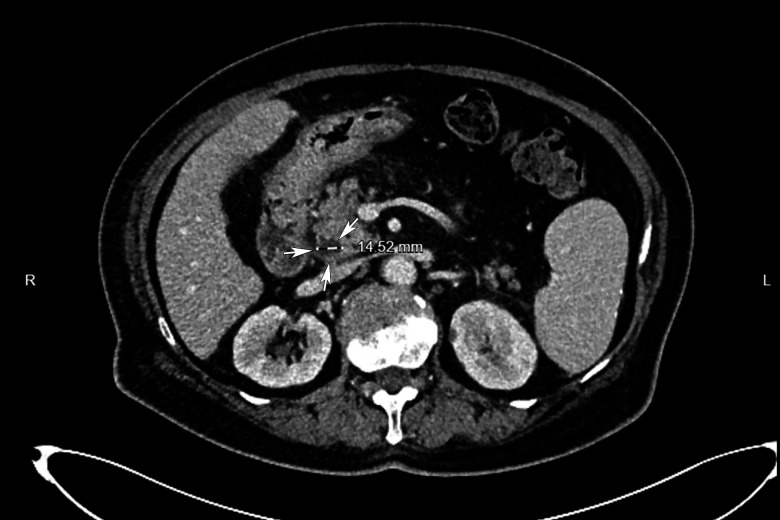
Enhanced abdominal CT scan. Coronal slice obtained through the pancreatic head and CBD. The CBD was dilated (white arrowheads) without PAD showing.
